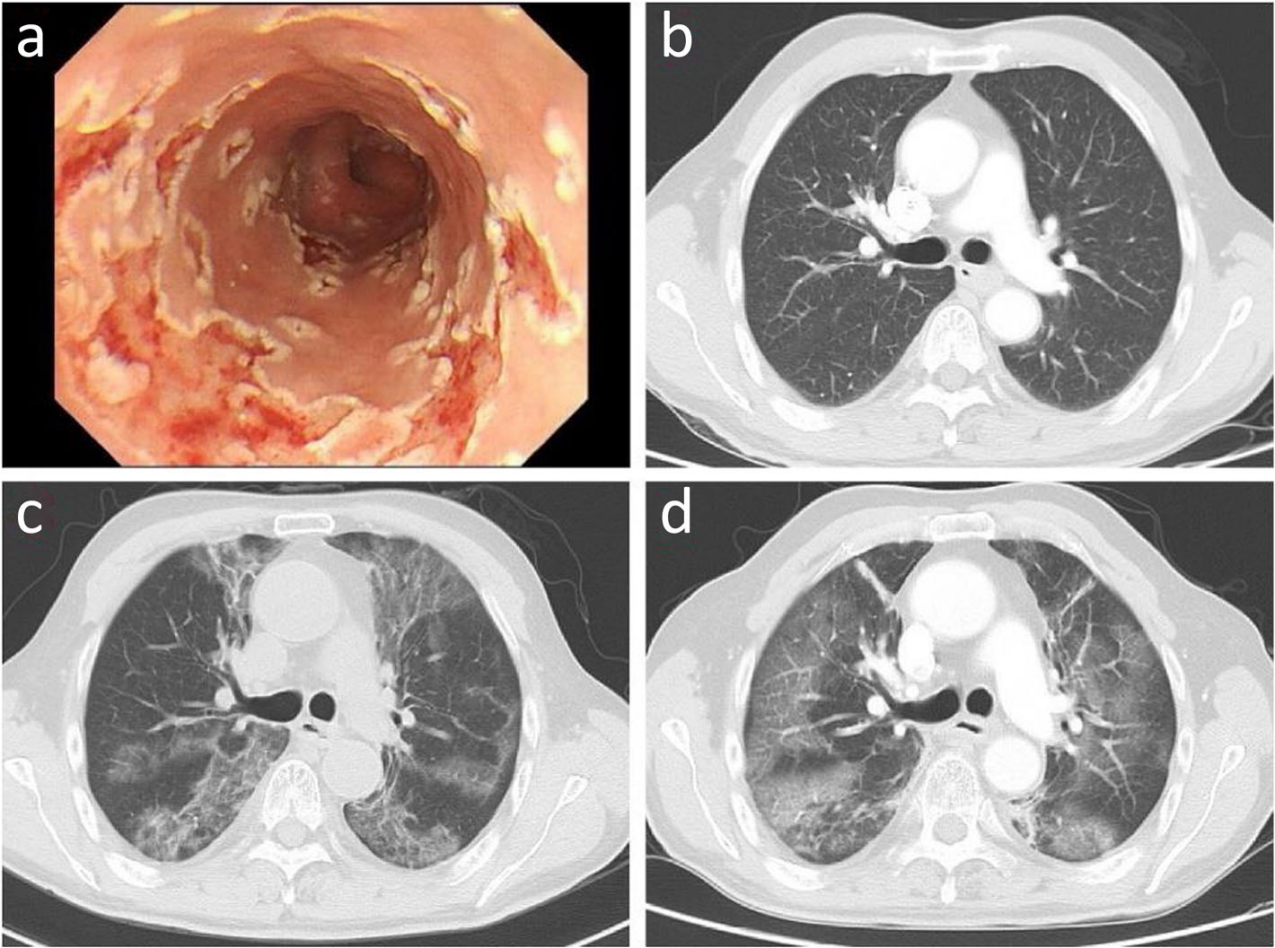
This 64-year-old esophageal cancer patient suffered grade 4 severe acute radiation-induced esophagitis (SARE) after only 10 fractions of radiotherapy. And he developed symptomatic radiation-induced pneumonitis (SRP) only 4 days after radiotherapy (RT) and then got infected of pneumocystis carinii during RT treatment. a: Endoscopy reveals diffuse mucosal erosion, sloughing, ulceration, and hemorrhage, and the endoscopic secretion culture revealed the fungal infection. b: Computed tomography (CT) image before radiotherapy; c: CT image that 6 days after radiotherapy; d: CT image that got infected of pneumocystis carinii during RP treatment.
